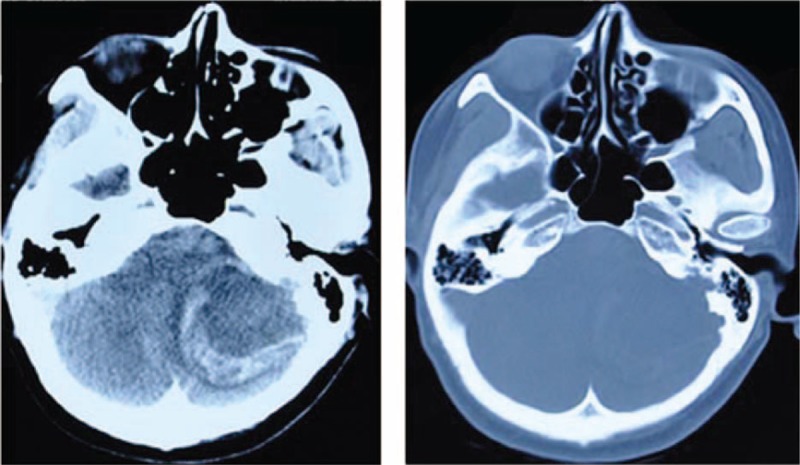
Emergent CT images showed a mass (about 4.9 × 4.3 cm) in the left cerebellum with surrounding bleeding and edema. There was a destruction of occipital bone close to tumor.
